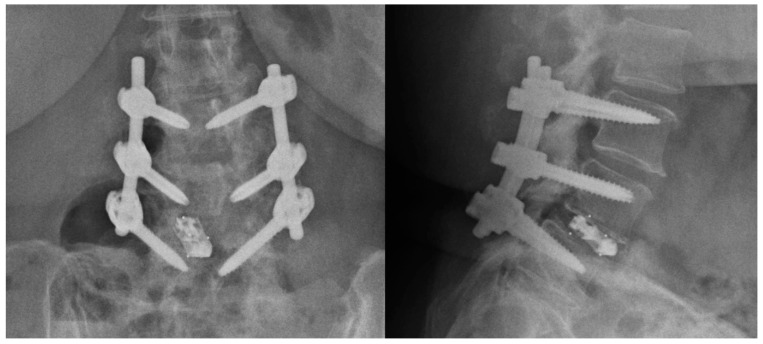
Anterior–posterior and lateral radiographs of the implants were taken a few days after the CT scan and showed intact pedicle screws.
